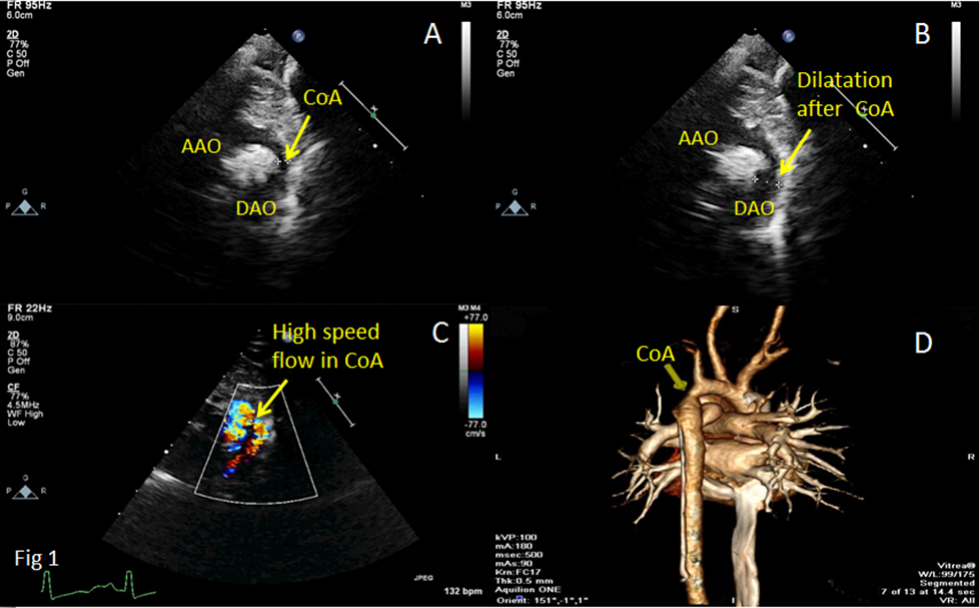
Transthoracic echocardiograms in suprasternal view of a 2-month-old boy showing[A] the location of the coarctation of aorta (CoA) between the ascending aorta (AAO) and descending aorta (DAO), [B] dilatation of the aorta after the CoA, and [C] high speed flow in the CoA. Computed tomographic angiography [D] obtained at 5 months of age confirmed the location of the CoA.
